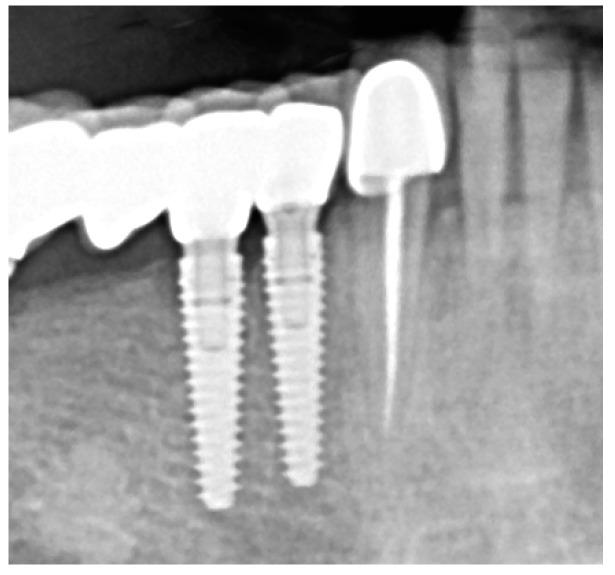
Implant without radiographic signs of crestal bone loss.
